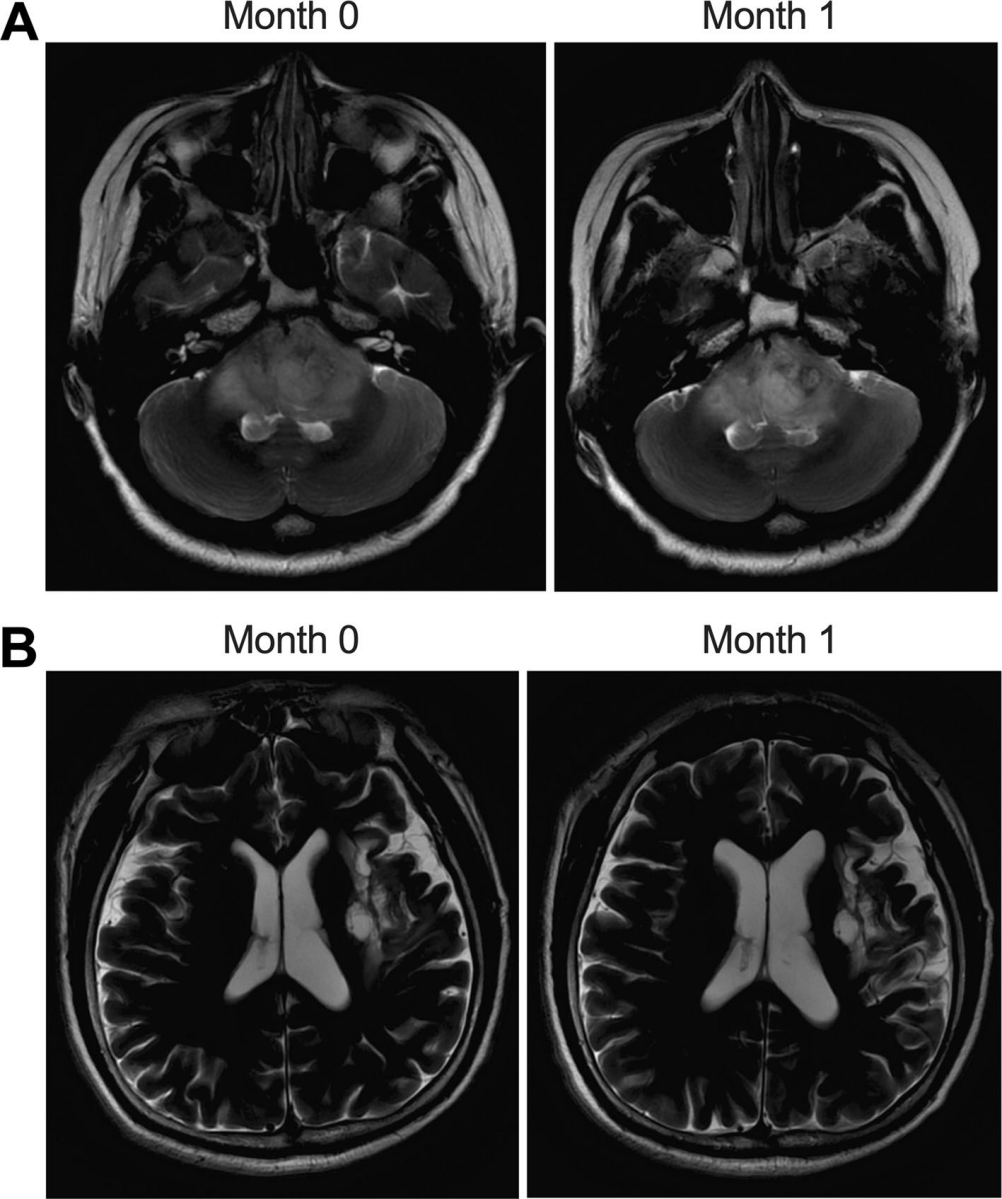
MRI results of brain injury were similar before and after RT. (A): Brain injury detected by MRI at Month 0; (B): Brain injury detected by MRI at Month 1.
